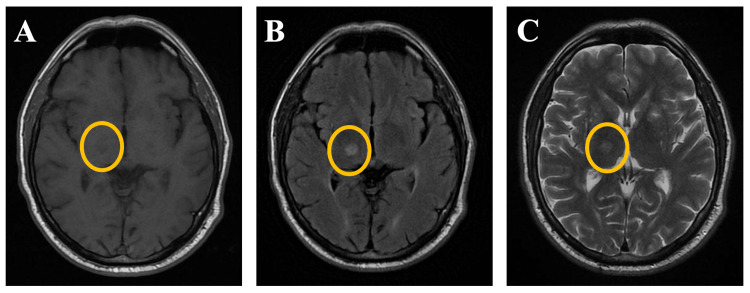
Non-contrast MRI brain images A) Axial T1 section with chronic right-sided lacunar infarct, B) FLAIR (Fluid Attenuated Inversion Recovery) showing right-sided lacunar infarct, and C) Axial T2 showing chronic right-sided lacunar infarct
